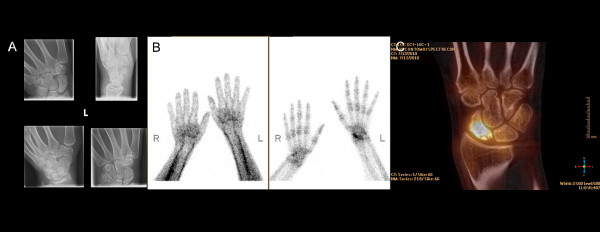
A 28-year-old male with suspected RC osteoarthritis after scaphoid fracture 6 years ago. The patient has a consolidated pseudoarthrosis of the scaphoid bone and persistent wrist pain. (A) Plain radiographs show focal sclerosis of the proximal pole of the scaphoid. (B) Planar bone scan revealed uptake in the scaphoid bone on late-phase images (left: early phase, right: late phase). (C) Coronal SPECT/CT images showed uptake in the proximal scaphoid (osseous remodeling) and a vital proximal scaphoid fragment. The patient received revisional osteosynthesis of the scaphoid.
